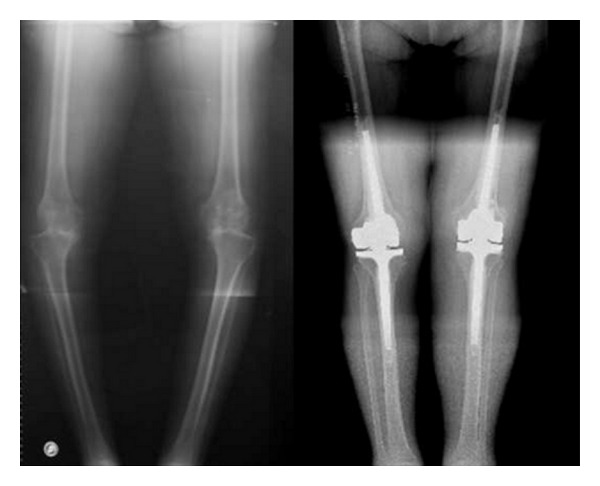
Preoperative X-ray of a patient with a severe bilateral varus deformity. Postoperative image of bilateral prosthesis showing the axial correction.
